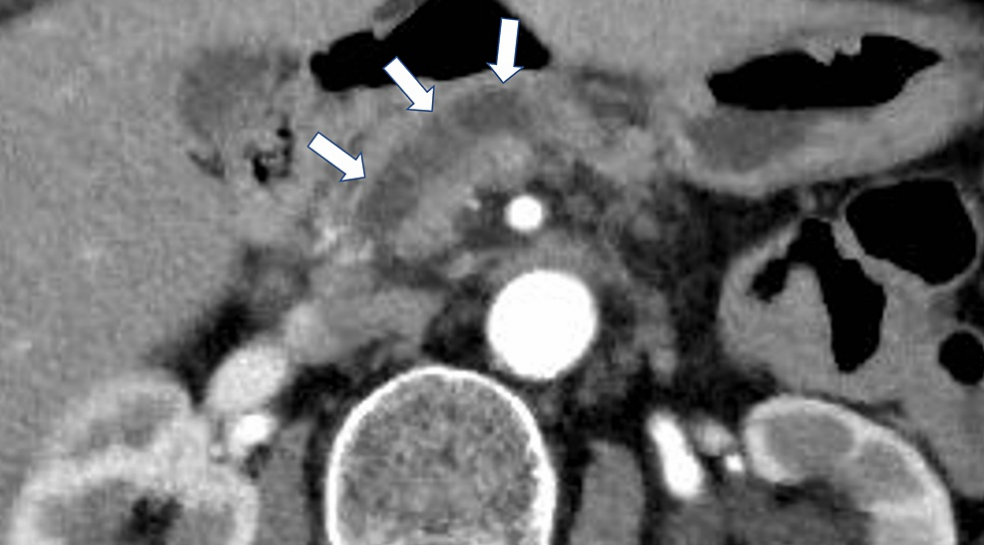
Dynamic CT showed dilatation of the main pancreatic duct (white arrows).
